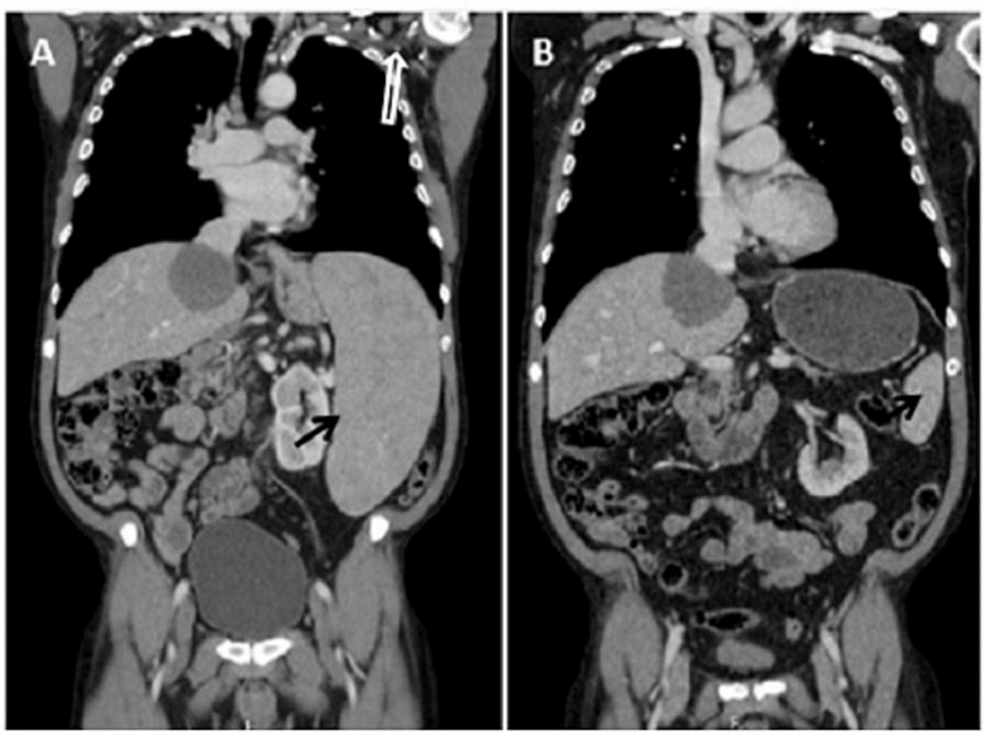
Thoraco-abdominopelvic CT scan (coronal plane comparison) A - at diagnosis; B - after R-CHOP chemoimmunotherapy. The black arrow highlights the enlarged spleen pre-treatment (A) and the normalization of its size after six cycles of chemotherapy (B). In the white arrow, it is possible to observe left axillary lymphadenopathies, absent after treatment.
